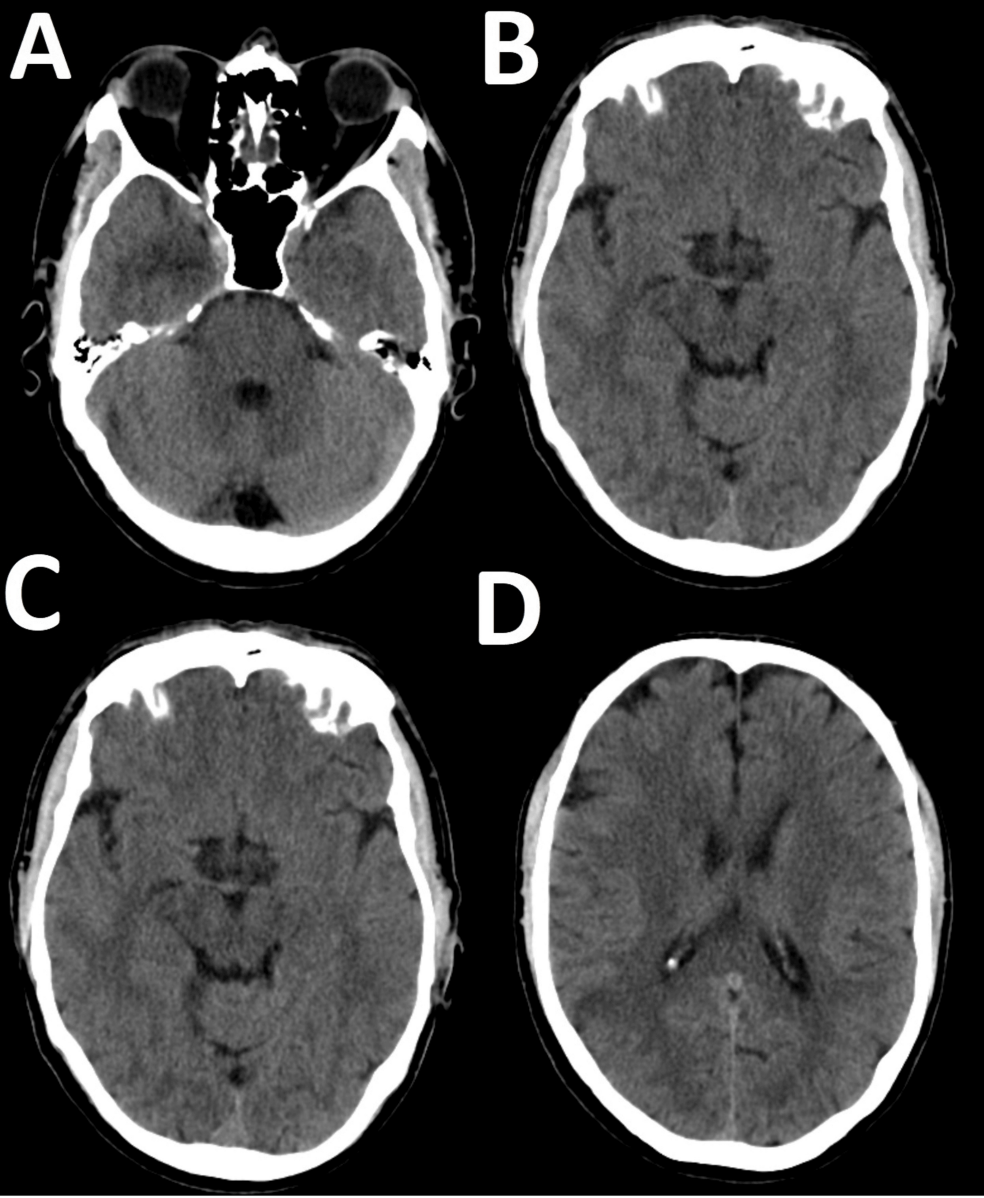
Computed tomography (CT) scan of the head showing no intracranial haemorrhage. (A) Posterior fossa; (B) midbrain; (C) thalamus and temporal lobes; (D) lateral ventricles and centrum semiovale
